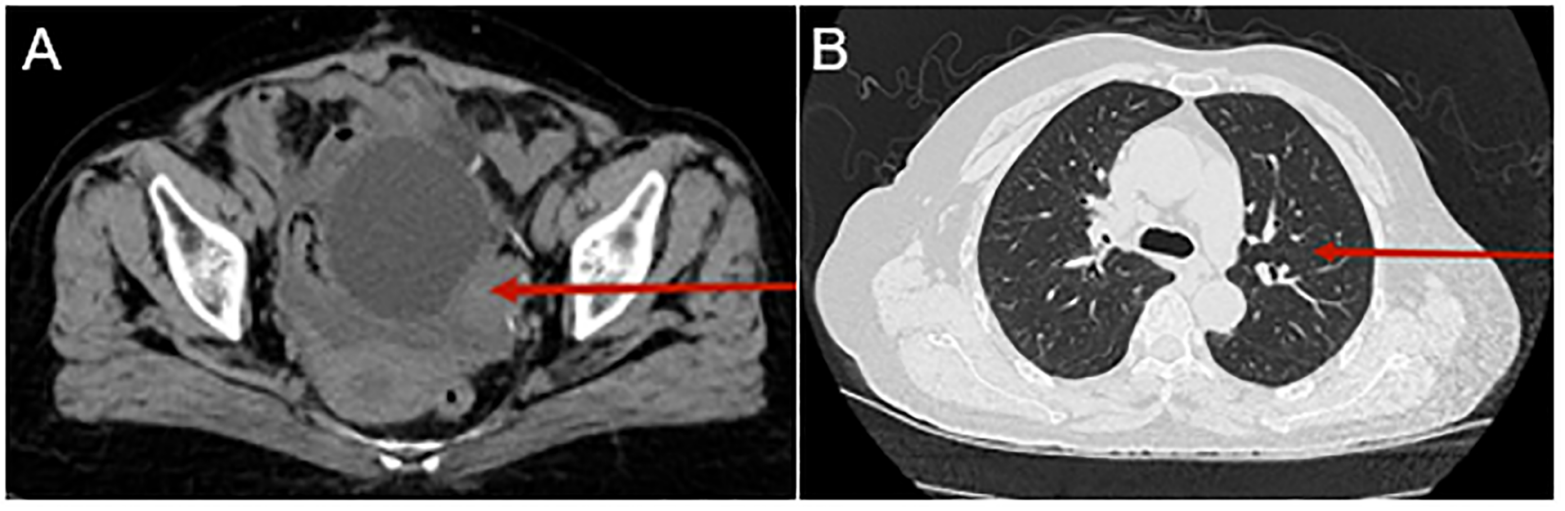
Computed tomography (CT) urography and chest CT following treatment with Tislelizumab. CT urography reveals a mass in the left posterior aspect of the bladder, which has grown since the last scan. The mass is indistinctly bordered by the left posterior wall of the bladder and the left adnexa [as indicated by the red arrow, (A)]. The chest CT scan also shows several little nodules in the middle and upper lobes [as shown by the red arrow, (B)].
